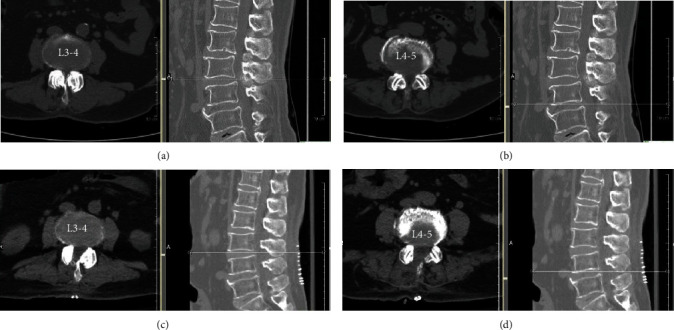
Preoperative computed tomography of L3-L4, L4-L5 stenosis (a, b), and postoperative computed tomography (c, d) obtained in one patient undergoing ULBD for 2-level stenosis.
